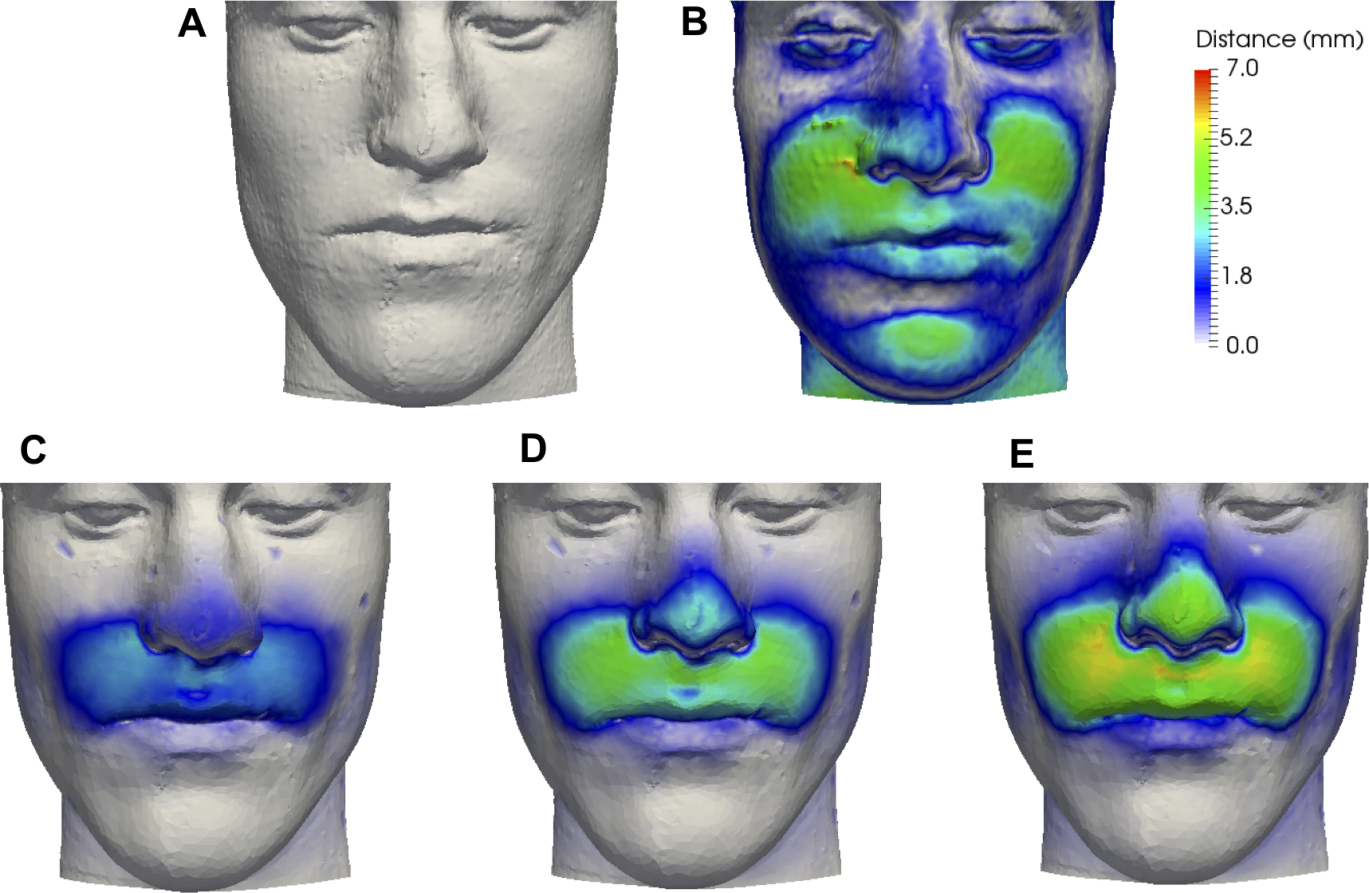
Patient 1 soft tissue prediction minimum and maximum. (A) Overall minimum soft tissue prediction displacements with *G*_*soft*_ = 94.4%, *E*_*soft*_ = 0.1 MPa, *ν*_*soft*_ = 0.45, *E*_*cart*_ = 0.5 MPa, *x*_*disp*_ = 3 mm, and (B) maximum soft tissue prediction displacements with *G*_*soft*_ = 30.5%, *E*_*soft*_ = 1 MPa, *ν*_*soft*_ = 0.49, *E*_*cart*_ = 5 MPa, *x*_*disp*_ = 7 mm. Blue depicts no change from the postoperative CBCT (0 mm), bright red depicts a maximum change (6 mm).
